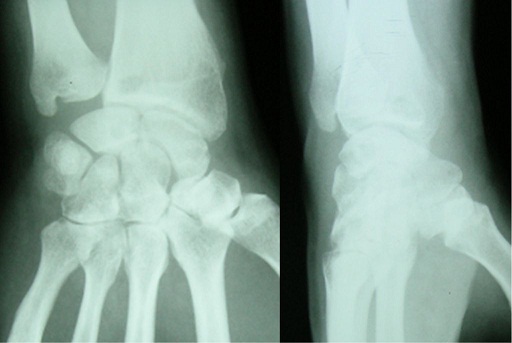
Radiographs showed consolidation after three months
